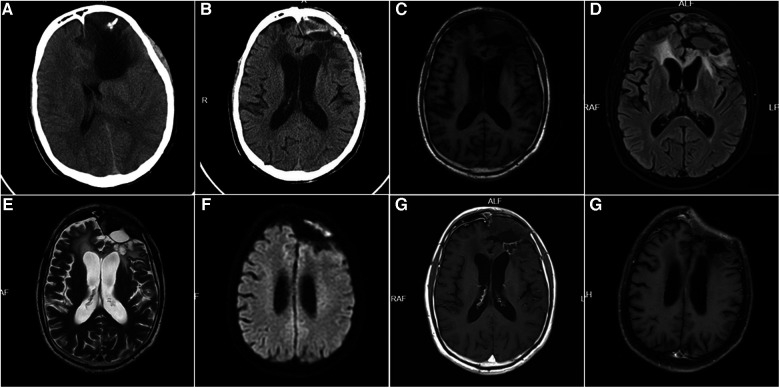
(A): A 60-year-old male patient with CT: left frontal glioma who underwent craniotomy for tumor resection. Postoperative pathology revealed diffuse astrocytoma and postoperative radiotherapy was performed. (B): 24 months after surgery, the patient had a headache accompanied by fever, and the skin flap showed no inflammation. CT showed the left frontal epidural lesion. (C): T1-weighted MRI showed an epidural hypointense and isointense lesion in the left frontal lobe. (D): FLAIR sequence of MRI showed epidural mixed signal lesions in the left frontal lobe. (E): T2-weighted MRI showed an epidural mixed signal lesion in the left frontal lobe. (F): Diffusion-weighted MRI (DWI) showed partial restricted diffusion in the left frontal epidural lesion. (G): Enhanced MRI showed an epidural lesion in the left frontal lobe with mild enhancement of the cyst wall. (H): The epidural abscess and the bone flap were removed. After 3 years of surgery, enhanced MRI reexamination showed no recurrence of epidural abscess.
